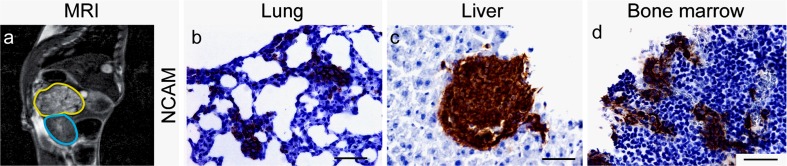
Neuroblastoma patient-derived orthotopic xenografts (PDOXs). a) Magnetic resonance imaging depicting a neuroblastoma PDOX (*circled in yellow*) located adjacent to the left kidney (*in blue*). Neuroblastoma PDOXs retain spontaneous metastatic capacity to distant organs including b lungs, c liver and d bone marrow, making them suitable models for studying and targeting human neuroblastoma metastasis. Metastatic cells are shown by expression of the neuroblastoma marker NCAM/CD56. *Scale bar* 50 μm
